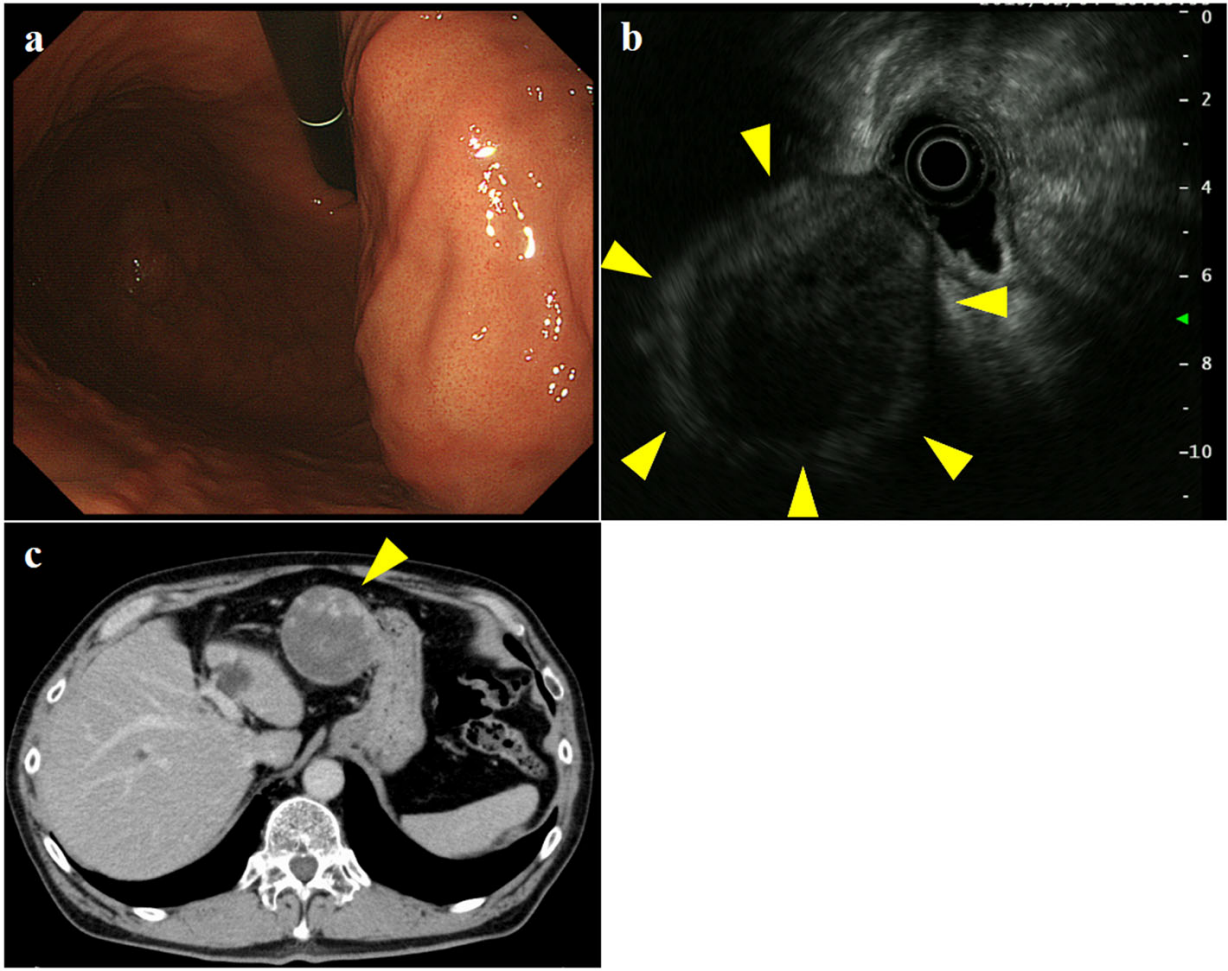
Findings of preoperative examination. Esophagogastroduodenoscopy (EGD) revealed a gently sloping protruding lesion with smooth surface mucosa, located anterior to the lesser curvature of the gastric body. Ultrasonic endoscopy (EUS) showed an extra-stomach hypoechoic tumor with a diameter of 79 × 58 mm that seemed to be connected to the smooth muscle layer of the stomach. Enhanced computed tomography (CT) revealed a solid tumor, 70-mm in diameter, which also seemed to be connected to the lesser curvature side of the gastric body
